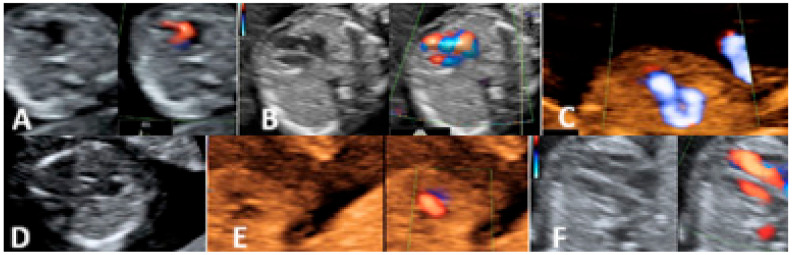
(A) Atrio-ventricular septal defect (Right side—Grey Scale and Left side—Color Doppler Mode). (B) Hipoplastic left heart ((Right side—Grey Scale and Left side—Color Doppler Mode) (C) Right aortic arch (Sepia-Color Doppler Mode). (D) Atrio-ventricular septal defect—Grey Scale) (E) Atrio-ventricular septal defect. (Right side—Sepia and Left side—Color Doppler Mode). (F) Hipoplastic left heart (Right side—Grey Scale and Left side—Color Doppler Mode).
